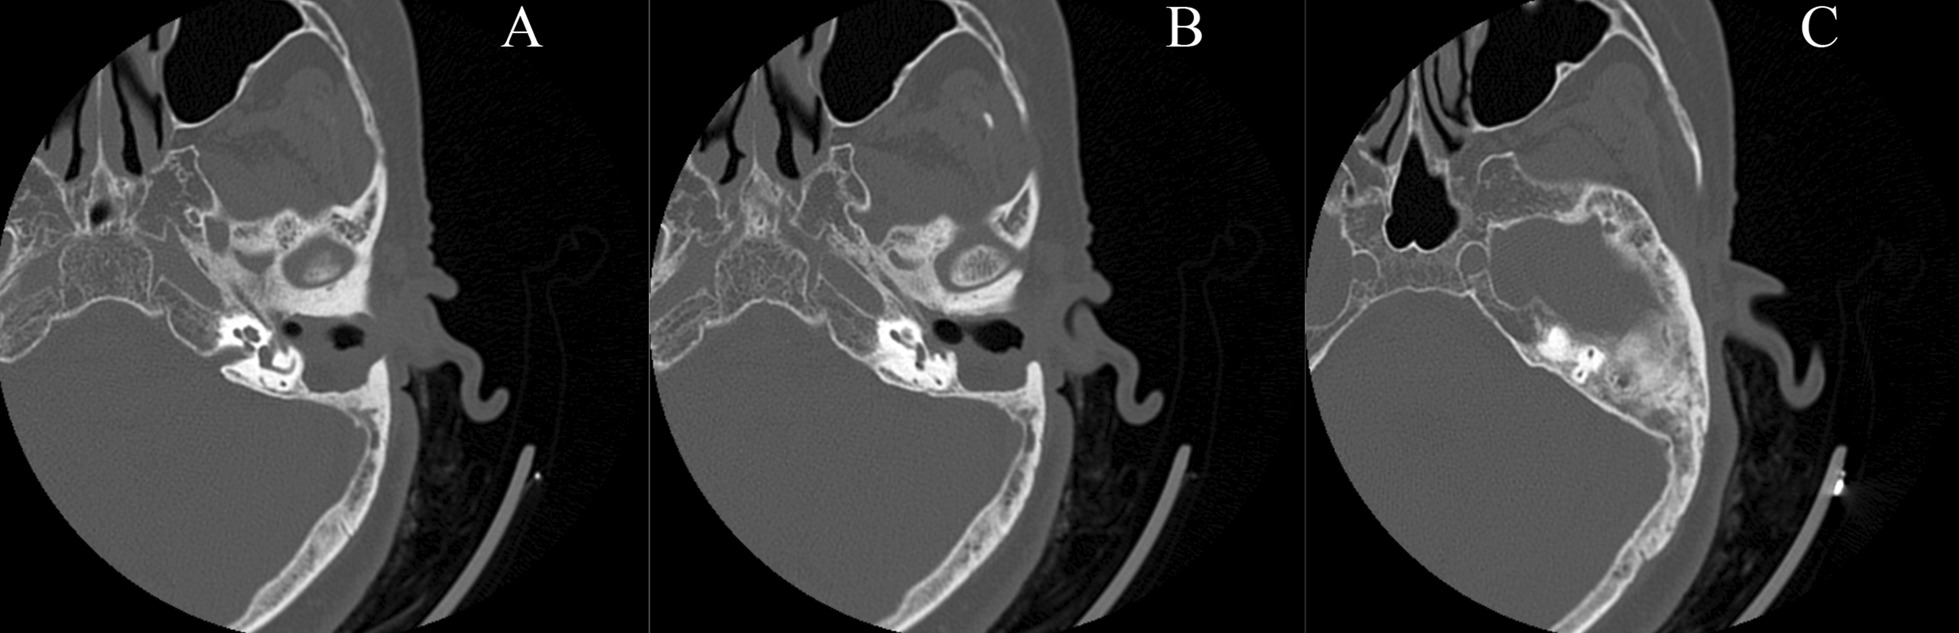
Computed tomography (a, b, c) revealed part of the left temporal bone
